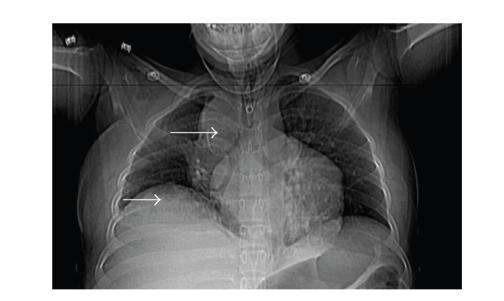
Chest X-ray. Right paratracheal soft tissue opacity measuring approximately
